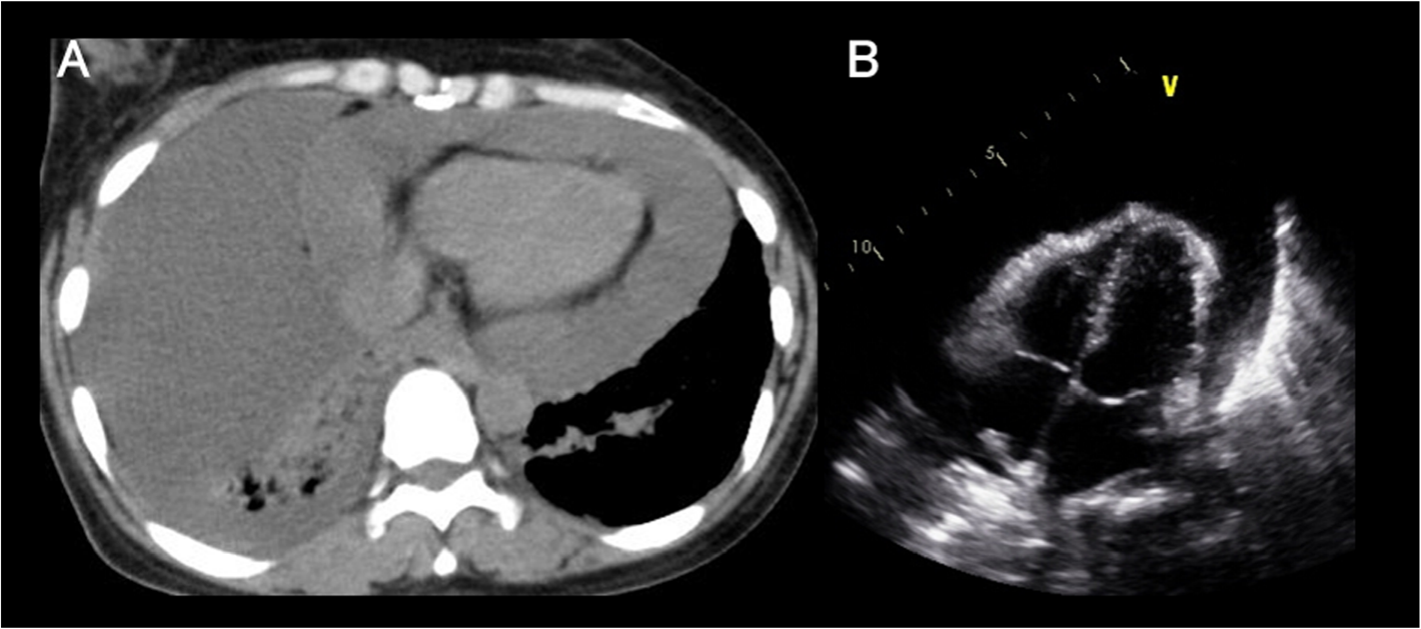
Radiologic and echocardiographic findings. a Chest computed tomography reveals right massive pleural effusion and pericardial effusion. b Echocardiography reveals massive pericardial effusion with right ventricle collapse
